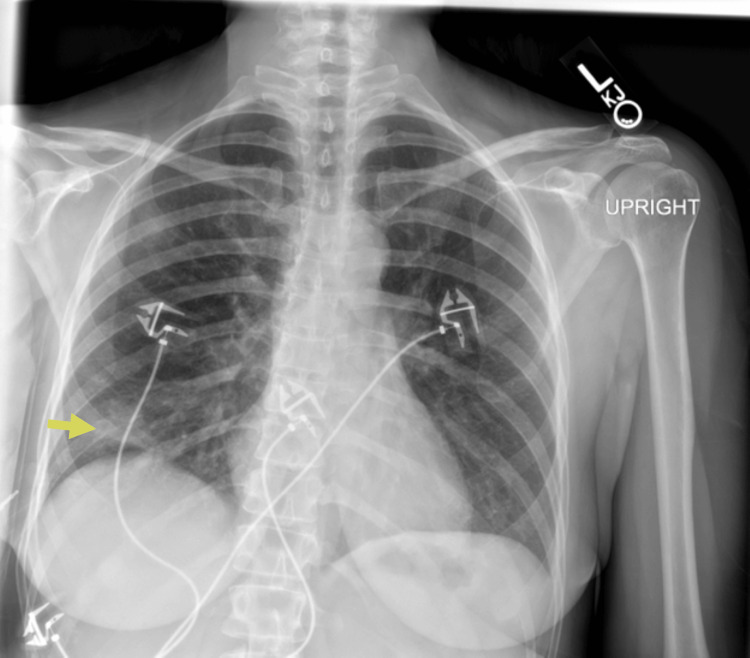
Chest radiograph showing right basilar opacities (yellow arrow)
